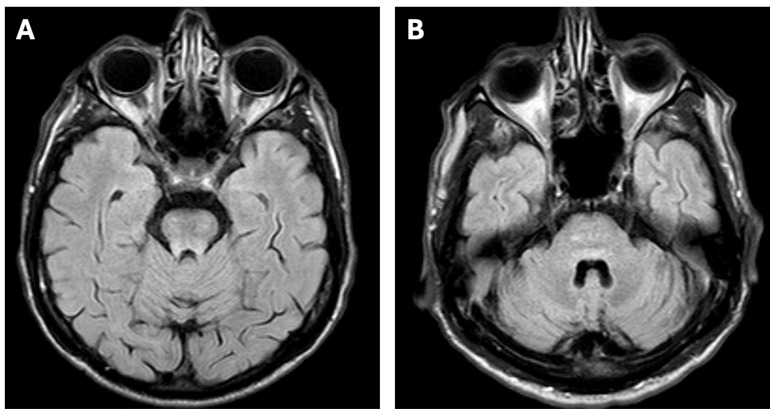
Brain MRI of the patient showing increased signal intensity in the pons (A) and midbrain (B) on the T2-weighted sequence.
